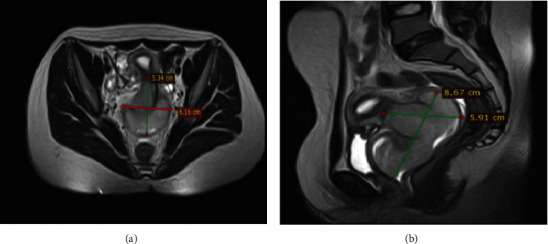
MRI of the primary tumor on (a) axial and (b) sagittal planes showed lobulated 8 *cm* × 6 *cm* × 5 *cm* well-defined homogeneous T2 hyperintense and T1 isointense cervical and vaginal mass expanding in the vaginal canal. Postcontrast imaging also showed a mild homogenous contrast enhancement suggestive of cervicovaginal rhabdomyosarcoma.
